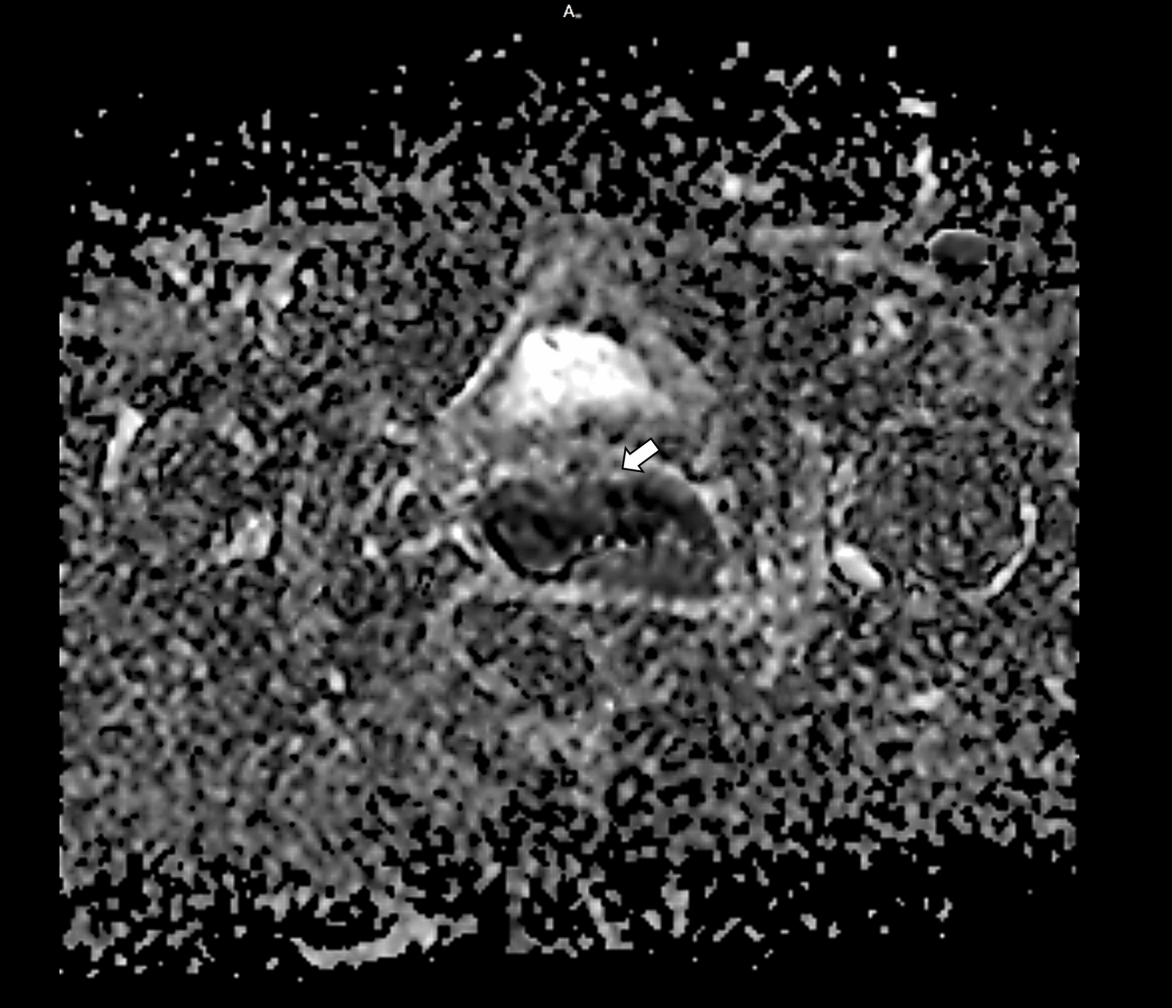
Diffusion-weighted imaging–apparent diffusion coefficient (DWI–ADC) maps show corresponding low ADC values within the lesion (arrow), confirming true restricted diffusion consistent with carcinoma of the cervix.
